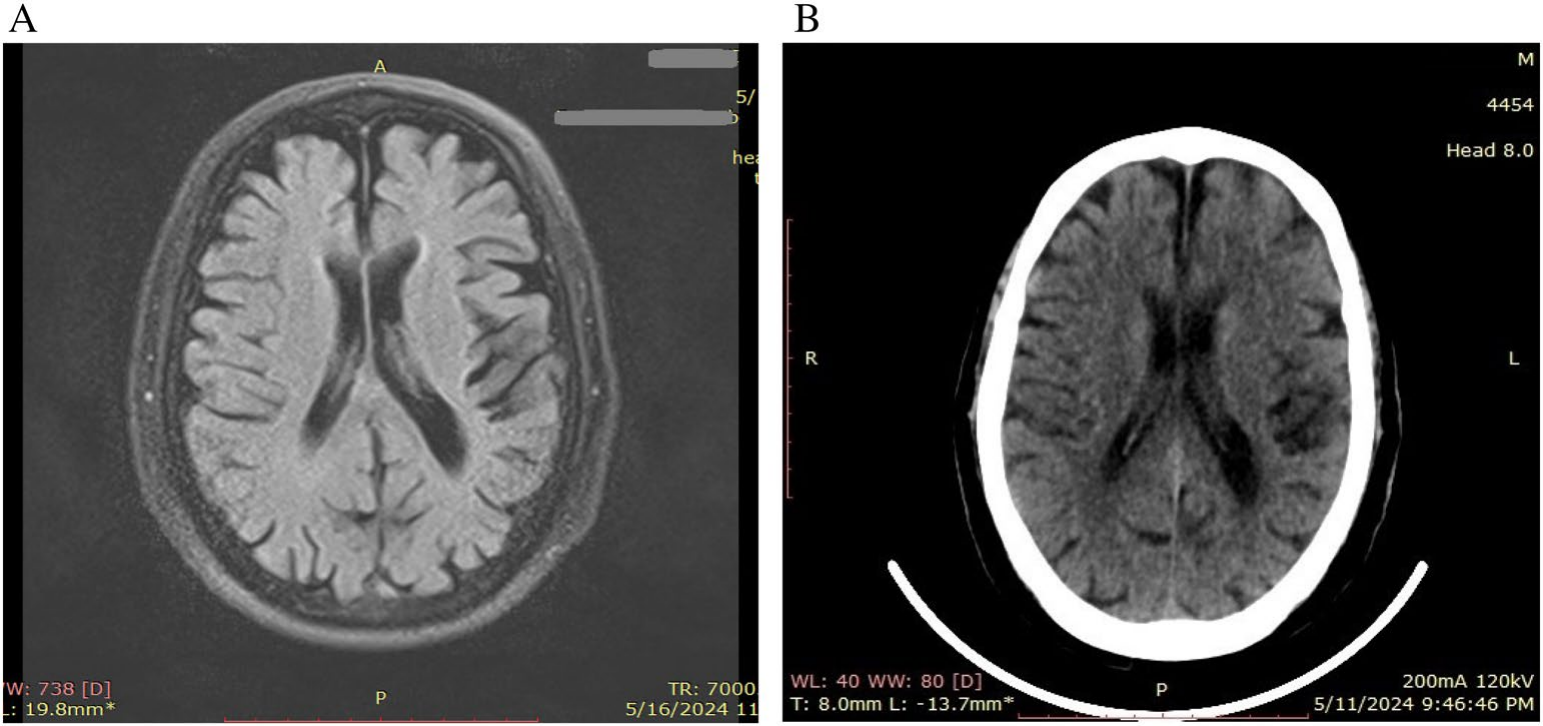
(A) Magnetic resonance imaging (MRI) of the head that was performed 7 days after admission shows gliosis and malacia at left frontoparietal lobes and cortical focus in the superior gyrus of the left frontal lobe in favor of acute ischemia. (B) Follow‐up brain computed tomography (CT) scan shows a hypodense area in the frontoparietal lobe, consistent with ischemic stroke and no evidence of intracranial hemorrhage.
